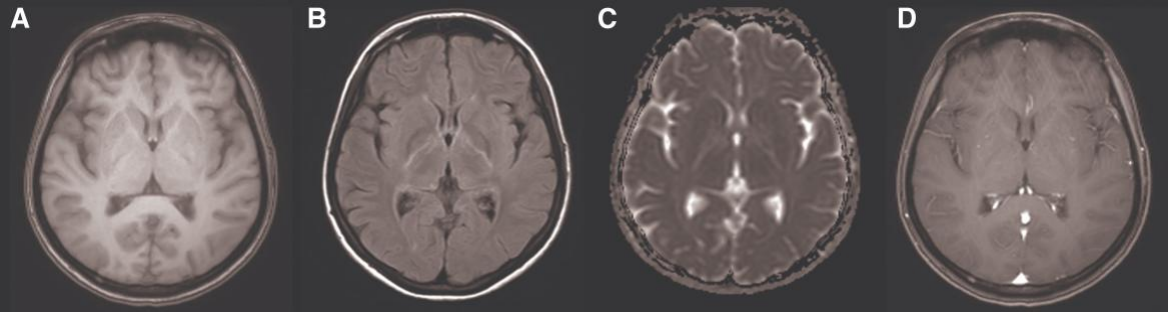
Brain MRI findings axial magnetic resonance imaging (MRI) performed on day X + 6 (the day of admission) and day X + 14 showed no space-occupying lesions, hemorrhage, or abnormal enhancement. FLAIR sequences from day X + 6 demonstrated subtle hyperintense signals in the bilateral posterior limbs of the internal capsules, corona radiata, pons, and midbrain. These findings were interpreted as possibly consistent with HIV-associated viral encephalitis. Images from left to right: (*A–C*) T1-weighted, FLAIR-T2, and ADC map (all acquired on day X + 6); (*D*) contrast-enhanced T1-weighted (T1 + Gad, acquired on day X + 14). Abbreviation: MRI, magnetic resonance imaging.
